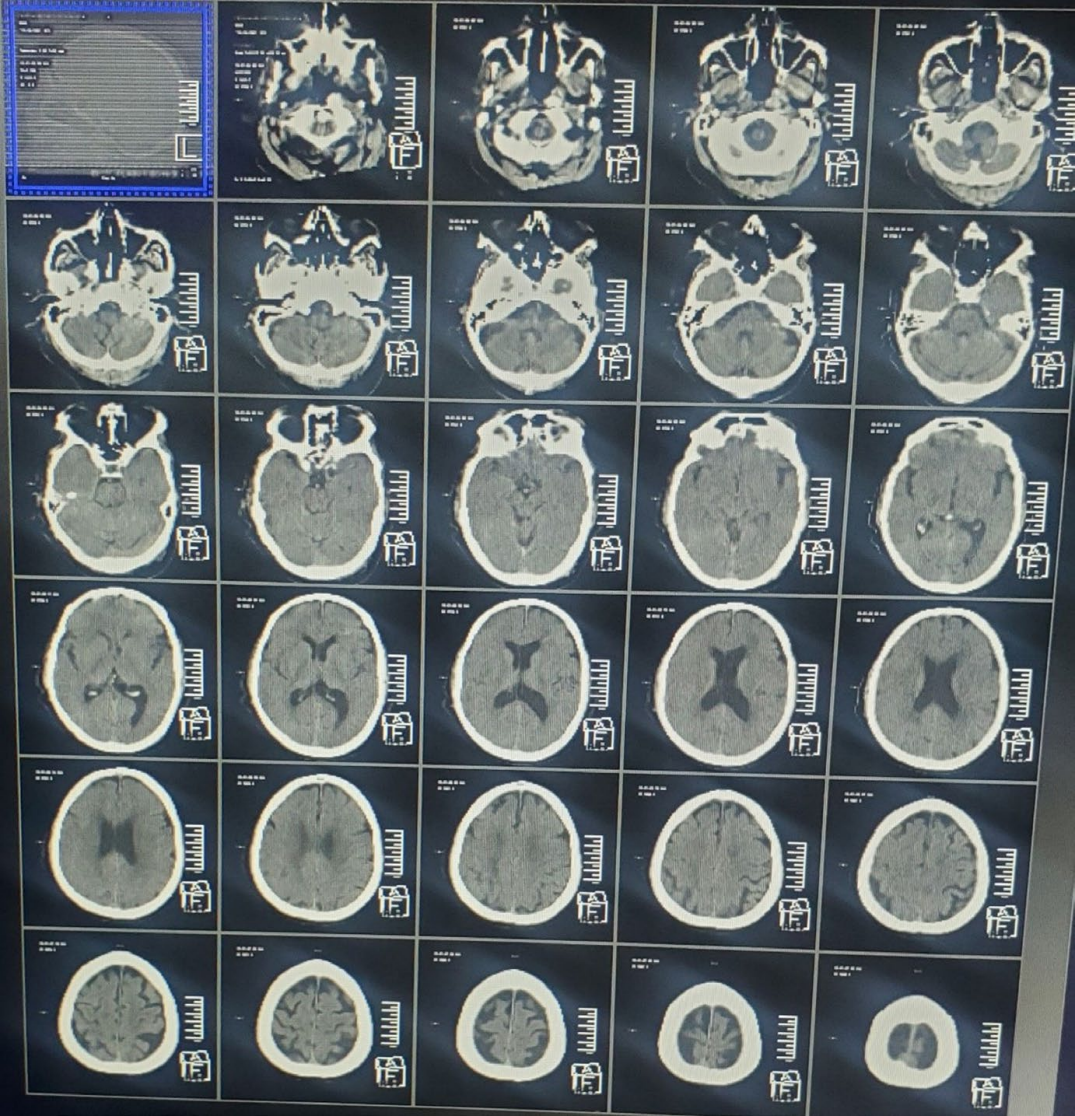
CT head showed no evidence of ischemic changes, intracranial hemorrhage, and space‐occupying lesions.
